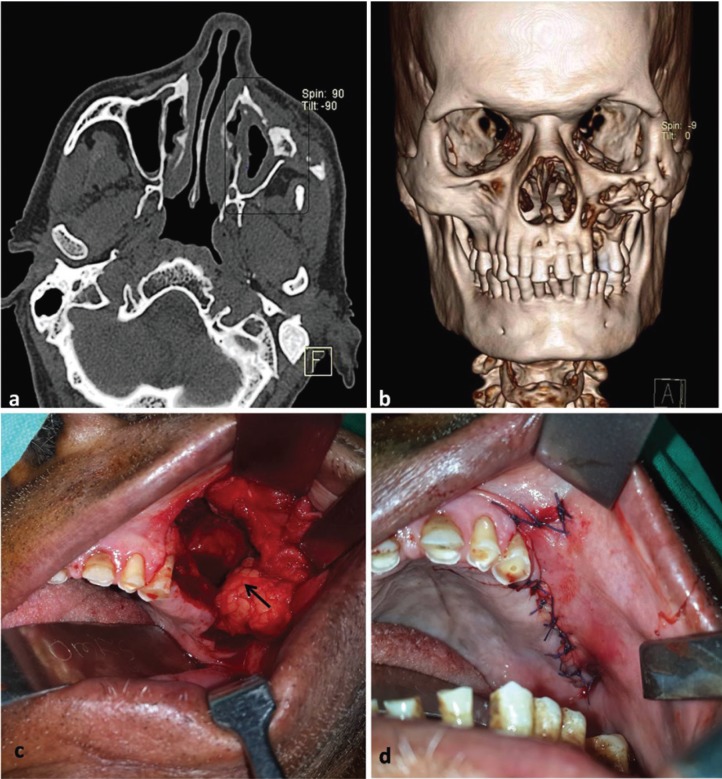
[Case 1] CT scan (axial section) showing thickening of antral lining and destruction of anterior wall of maxilla (a); Three dimensional formatted CT image showing involvement of left maxilla (b). Intraoperative pictures showing surgical debridement and removal of the sequestrum with the buccal fat pad mobilized into the defect (arrow) (c); closure of the oroantral communication (d).
